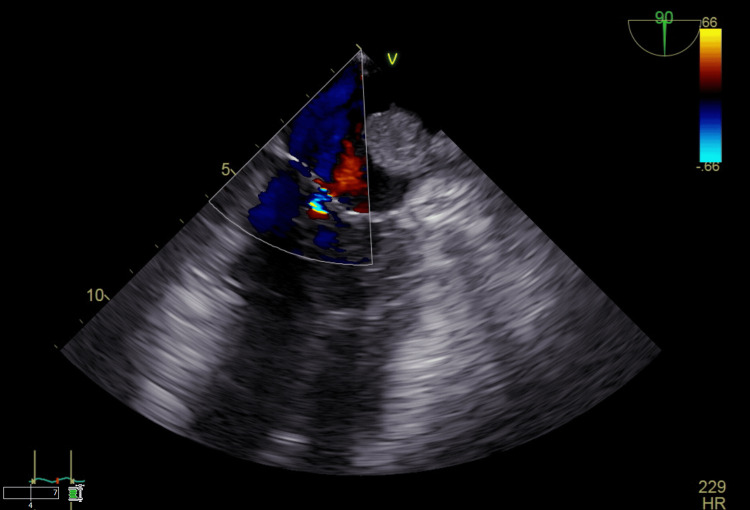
Transesophageal echocardiogram showing turbulent blood flow inside the left atrium
